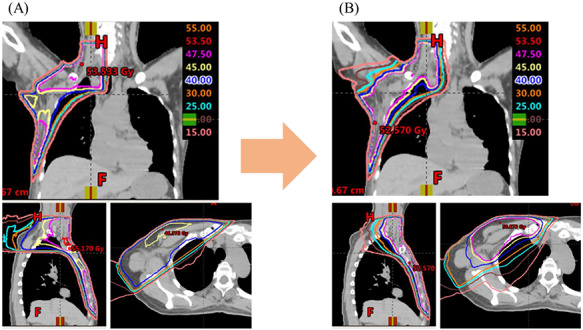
Comparison of distribution maps. (A) Axial CT image and (B) overhead view of beam setup. ‘Hybrid VMAT (H-VMAT)’ combines conventional tangential field mainly for the chest area and VMAT mainly for the supraclavicular area and marginal zone.
